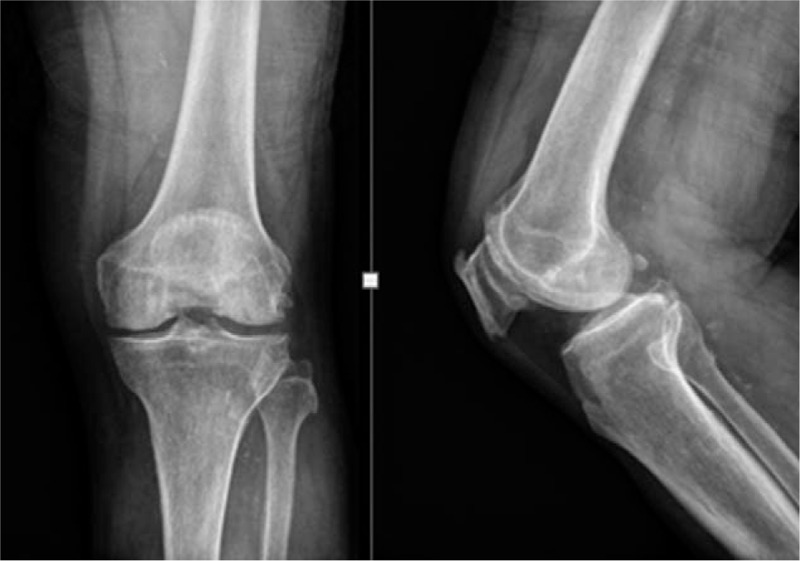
X-ray showed mild arthritic changes and no joint effusion.
